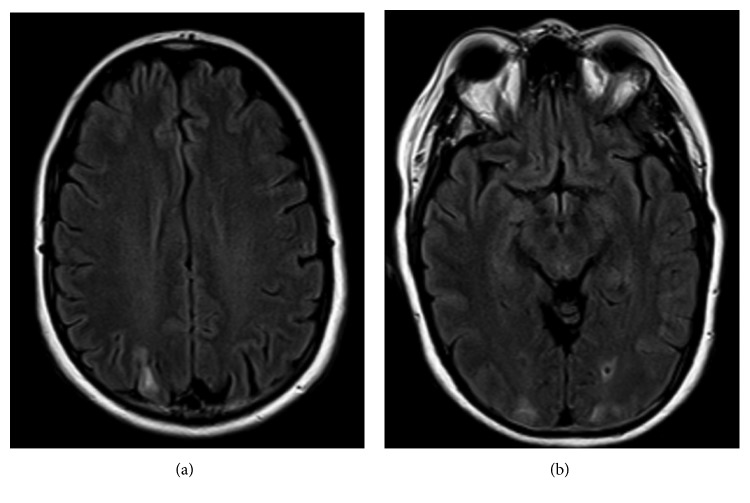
Axial FLAIR images of the brain demonstrating slight improvement of hyperintense signals in the (a) posterior right parietal lobe and (b) posterior occipital lobes bilaterally.
